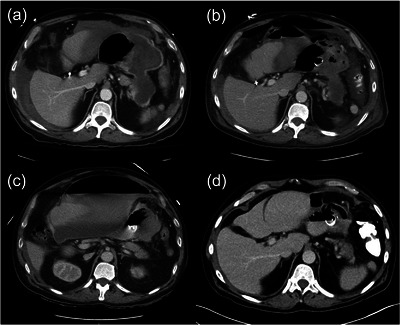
Serial computed tomography images (a) On admission, a peripancreatic pseudocyst with mild hemorrhagic ascites was observed. (b) On the third day, the pneumoperitoneum developed after lumen‐apposing metal stent cystgastrostomy. (c) On the ninth day, a new peritoneal fluid collection with free air became apparent. (d) Both the fluid collection and pseudocyst improved after percutaneous drainage on the seventeenth day.
